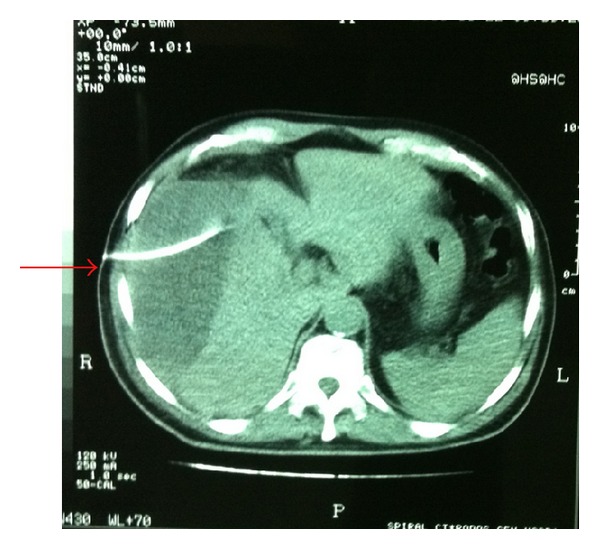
Percutaneous drainage of the collection under CT guidance (arrow).
